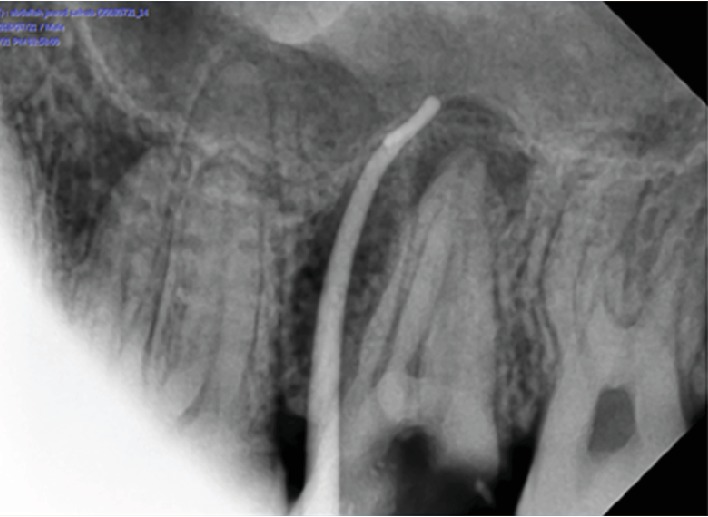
Periapical X-ray shows gutta-percha inserted through sinus.
